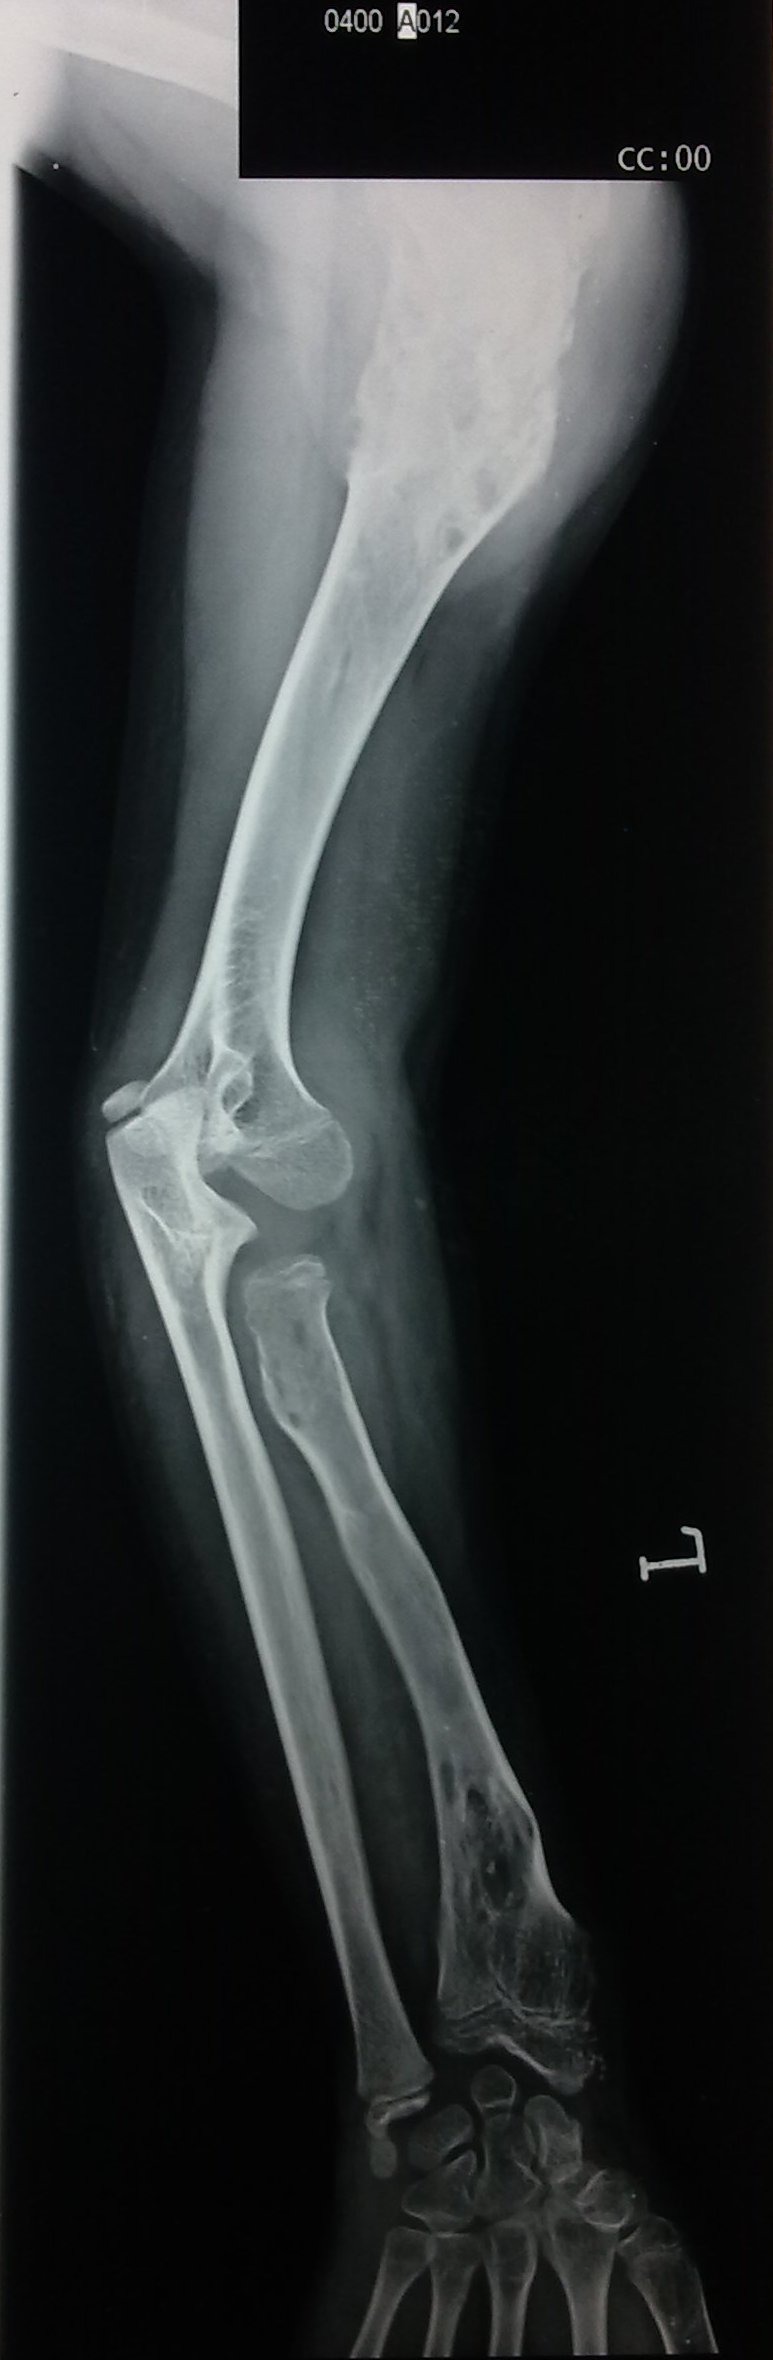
Figure 4: Plain radiograph - left upper limb: enchondromas involving proximal humerus and distal radius.
